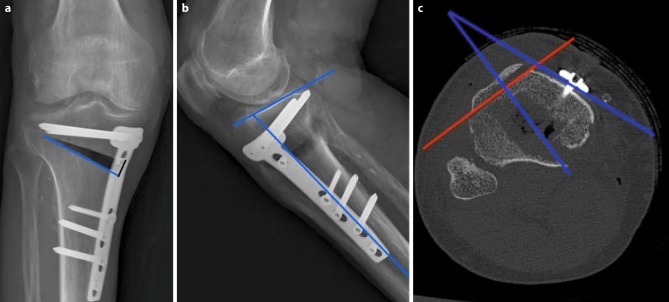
Measurement techniques on length of osteotomy (*blue line*) and medial osteotomy width (*black line*) are displayed in picture a; measurement technique of tibial slope and osteotomy slope (*blue lines*) are shown in picture b. In axial CT sequences, rotational offset of the plate (referring to the tibial tuberosity) was measured (*red lines*) (c)
